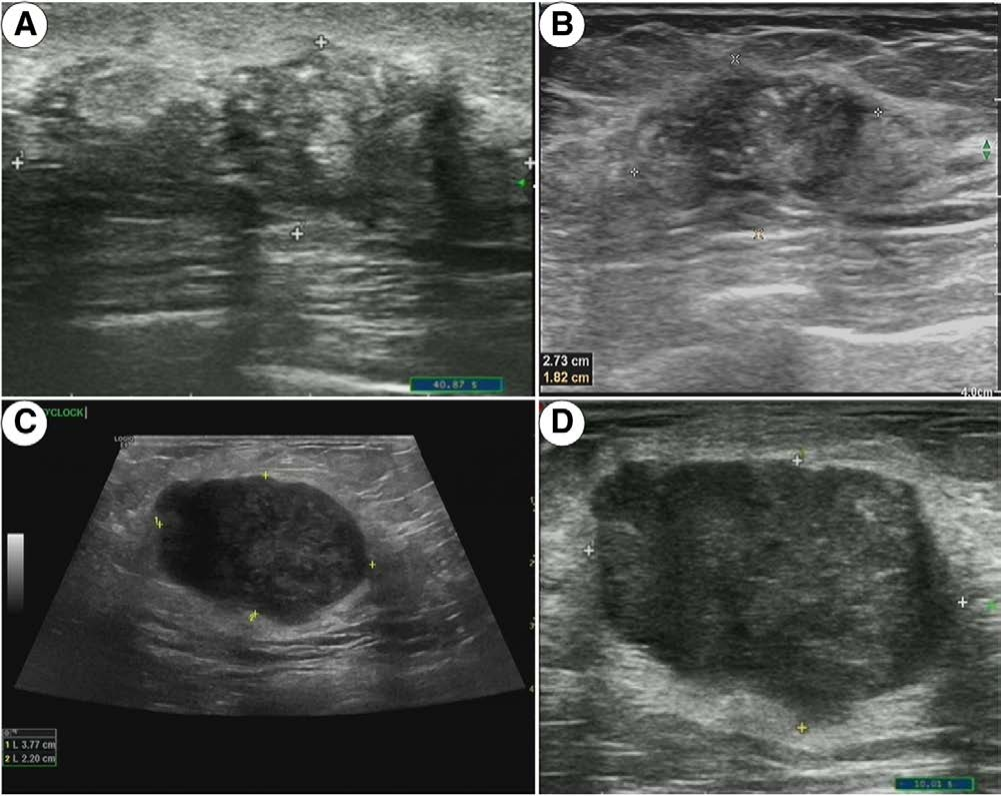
Ultrasound change of breast cancer before and after treatment of neoadjuvant chemotherapy. (A) Ultrasound image of breast cancer before neoadjuvant chemotherapy (NAC) in patients with pathological complete response (pCR), lesion size 43 mm × 15 mm; (B) ultrasound image of breast cancer after the second cycle of NAC treatment in patients with pathological complete response (pCR), lesion size 27 mm × 18 mm; (C) ultrasound image of breast cancer before neoadjuvant chemotherapy (NAC) in patients with non-pathological complete response (pCR), lesion size 38 mm × 22 mm; (D) ultrasound image of breast cancer after the second cycle of NAC treatment in patients with non-pathological complete response (pCR), lesion size 37 mm × 42 mm. NAC = neoadjuvant chemotherapy, pCR = pathological complete response.
